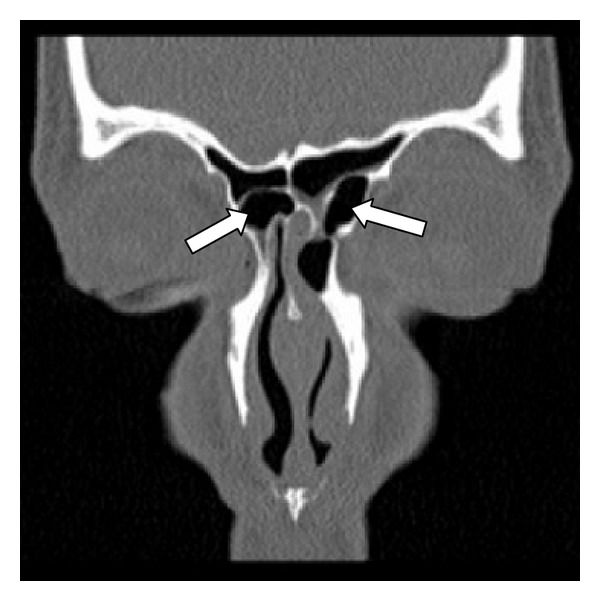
Type III frontal cells. A coronal CT scan showing bilateral type III frontal cells (arrows) pneumatizing from the frontal recesses into the frontal sinuses.
